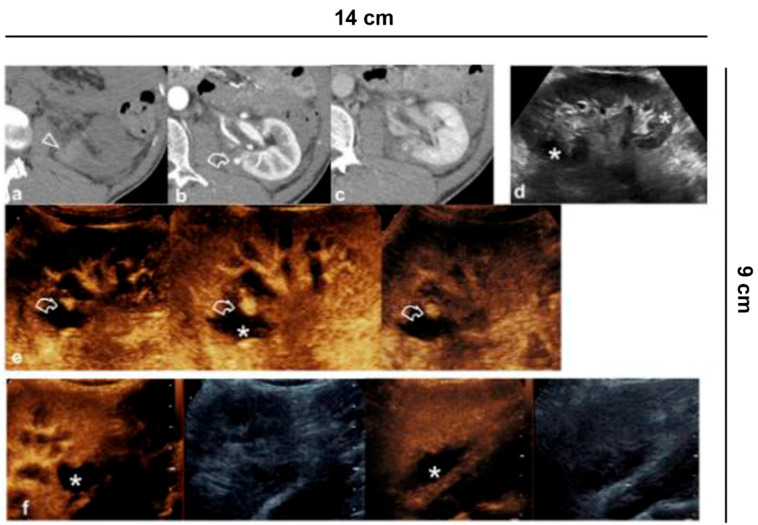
Vascular lesions. 35-year-old male patient with abdominal trauma treated conservatively. (a) CT without contrast medium shows parenchymal hyper-density from recent hematoma (arrowhead) at the lower pole of the left kidney; (b) A pseudoaneurysm (curved arrow) is recognized in the arterial phase, while in the (c) nephrographic phase the parenchymal contusion is more clearly recognized and the pseudoaneurysm is less visible; (d) B-mode US shows inhomogeneous echogenic areas (asterisks) corresponding to the contusions; (e) CEUS adequately shows all the vascular phases of the pseudoaneurysm (curved arrow) and the perirenal hematoma (asterisk); (f) Follow-up performed on 7th day.
